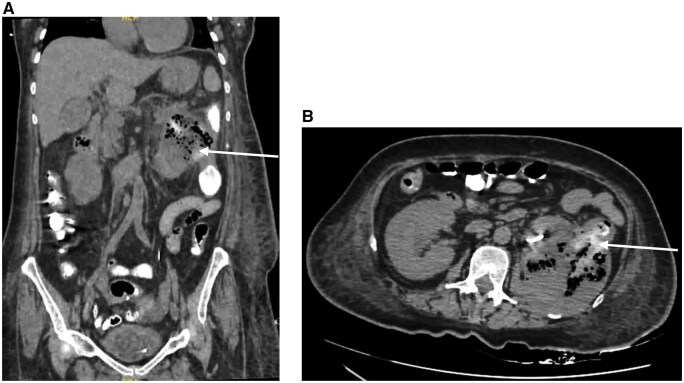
Coronal (A) and axial (B) CT images following rectal contrast administration demonstrating leakage of contrast (arrows) from the descending colon into the perinephric collection, confirming fistulous communication. Focal mural defect in the posteromedial wall of the descending colon (arrow in image B) corresponding to the site of fistulous communication.
